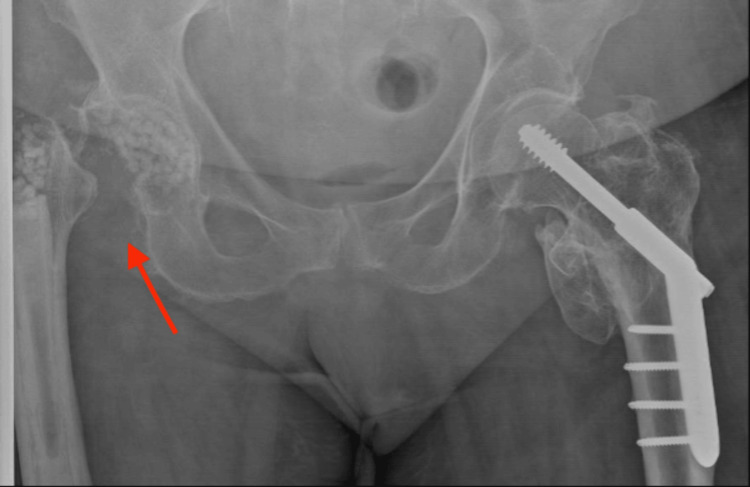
AP pelvis X-ray. The red arrow points to the right proximal femoral excision (Girdlestone procedure) with placement of antibiotic-eluting CSBs. AP: Anteroposterior; CSB: Calcium-sulphate bead
